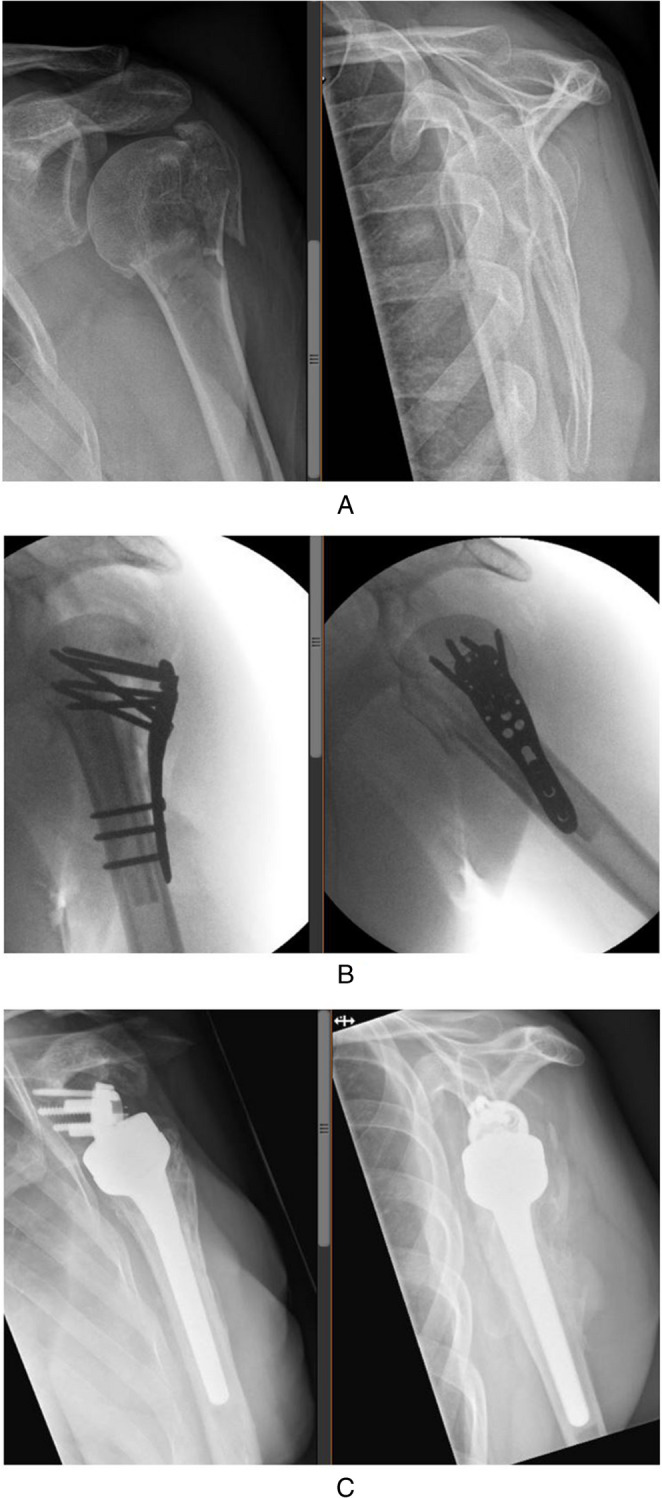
A 71 year old man with a 3-part valgus impacted PHF. A Fracture radiographic imaging. B Fluoroscopic images of osteosynthesis. C Radiographic imaging at final follow-up (18 months after RSA)
